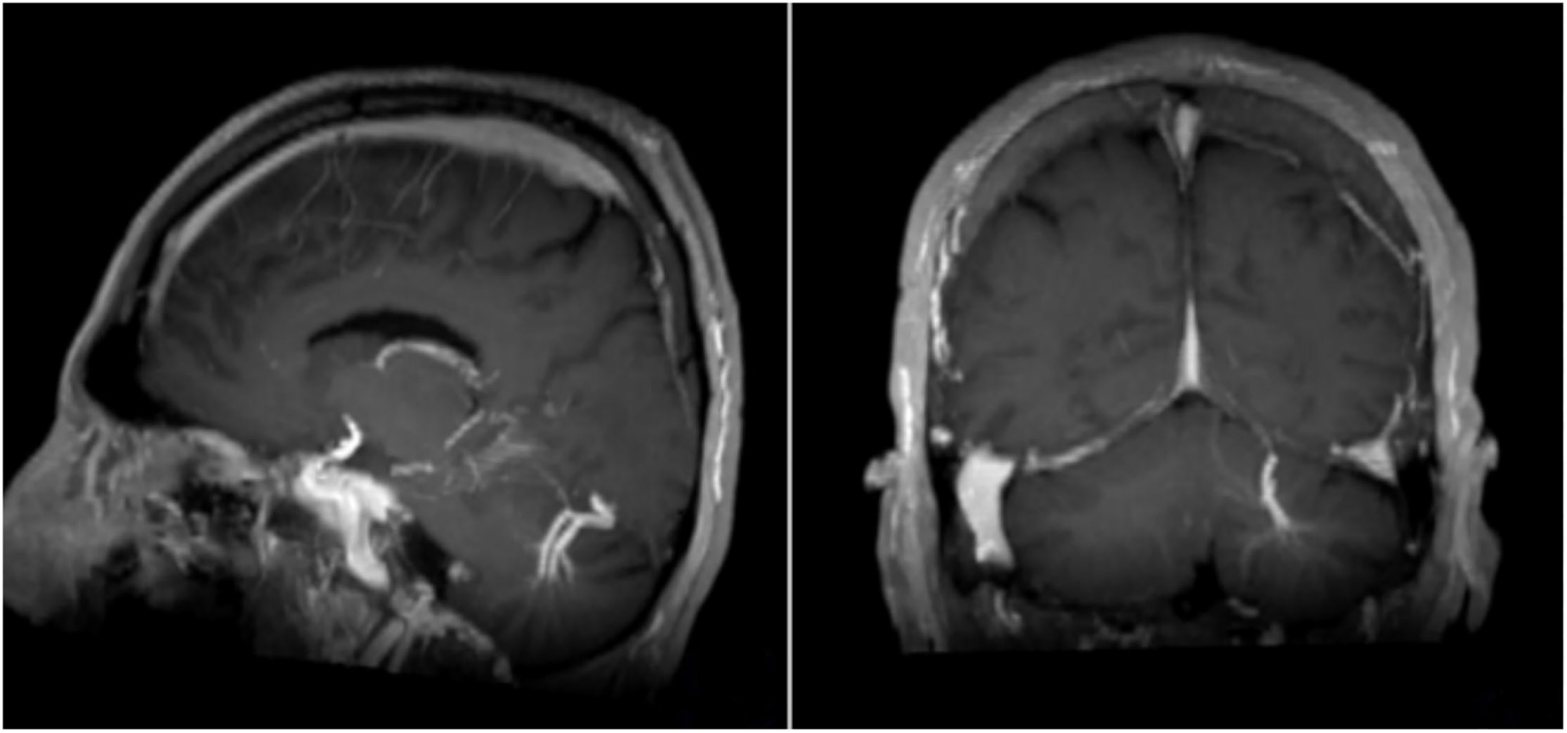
Sagittal and coronar T1-weighted MRI with intravenous contrast medium. Note the big DVA with two collecting veins cerebellar left.
